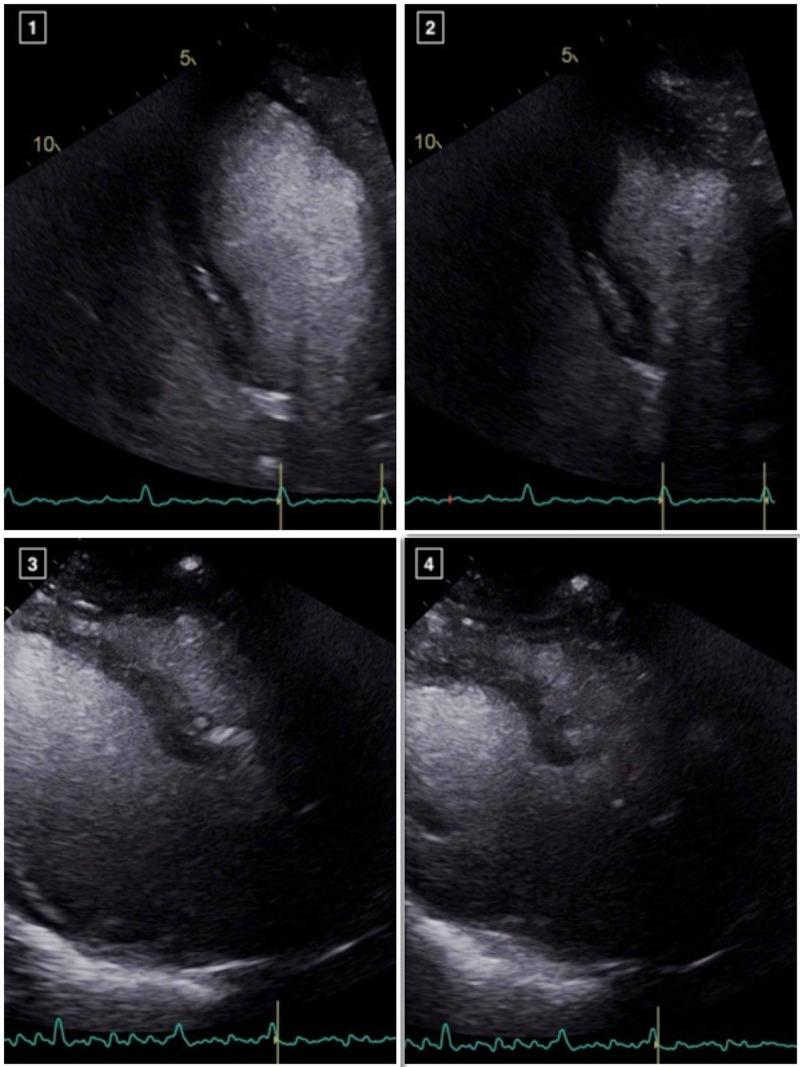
Apical 4-chamber views and parasternal long axis views demonstrating basal to mid LV systolic dysfunction with preserved distal and apical segments, in a reverse TakoTsubo pattern (images 1-4).
